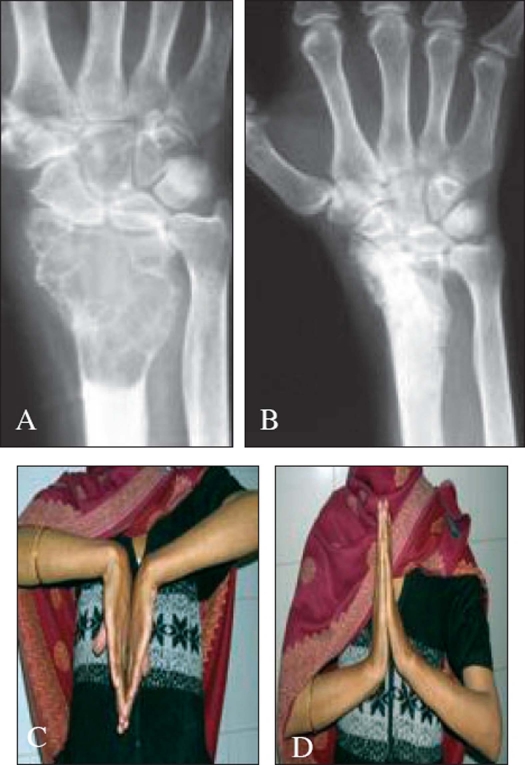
A) Pre-operative x-ray of recurrent GCT of distal end radius in a 35-year -old female. B) 8 month post-operative x-ray of the same patient. C), D) Clinical picture demonstrating good range of wrist movements in the same patient at 8 months follow up
